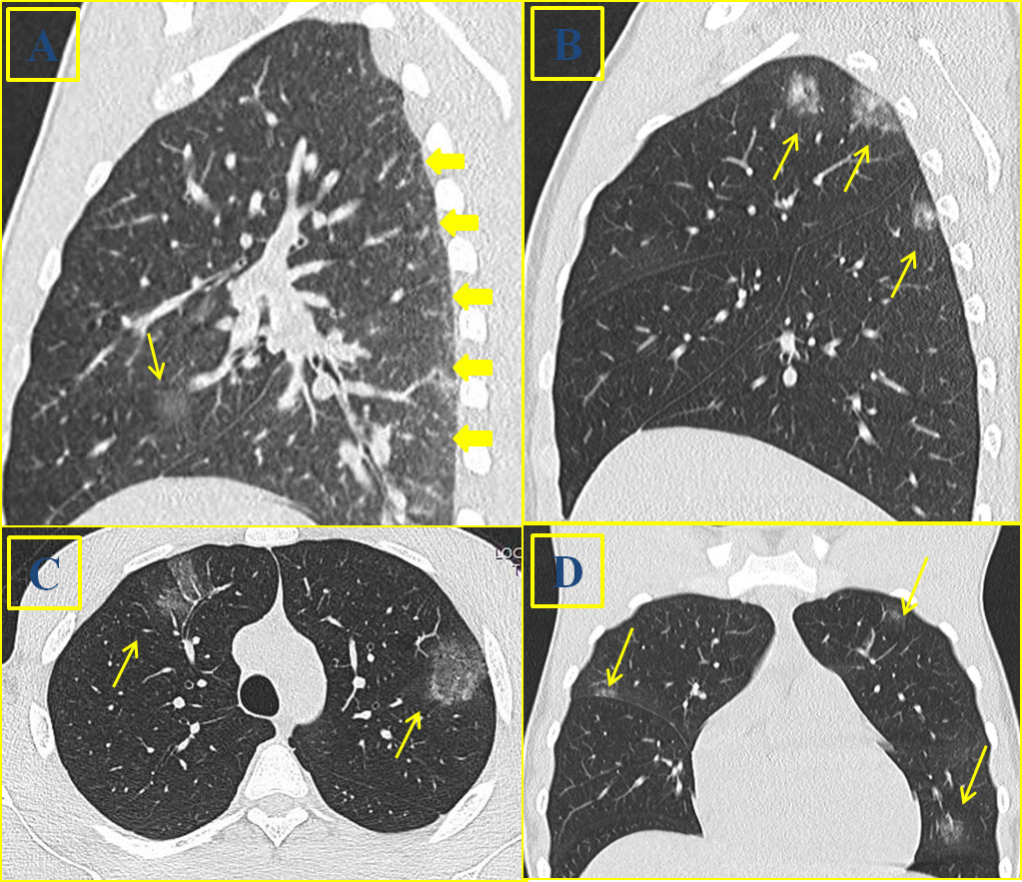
An 18-year-old male suffering from HBV hepatopathy. Multiple ground glass opacity of different size and density (arrows) can be identified close to the pleura at HRCT on sagittal (4A), axial (4C) and coronal (4D) images. Mixed nodular lesions with peri-nodular halo are detected on sagittal reconstructed image (4B)
